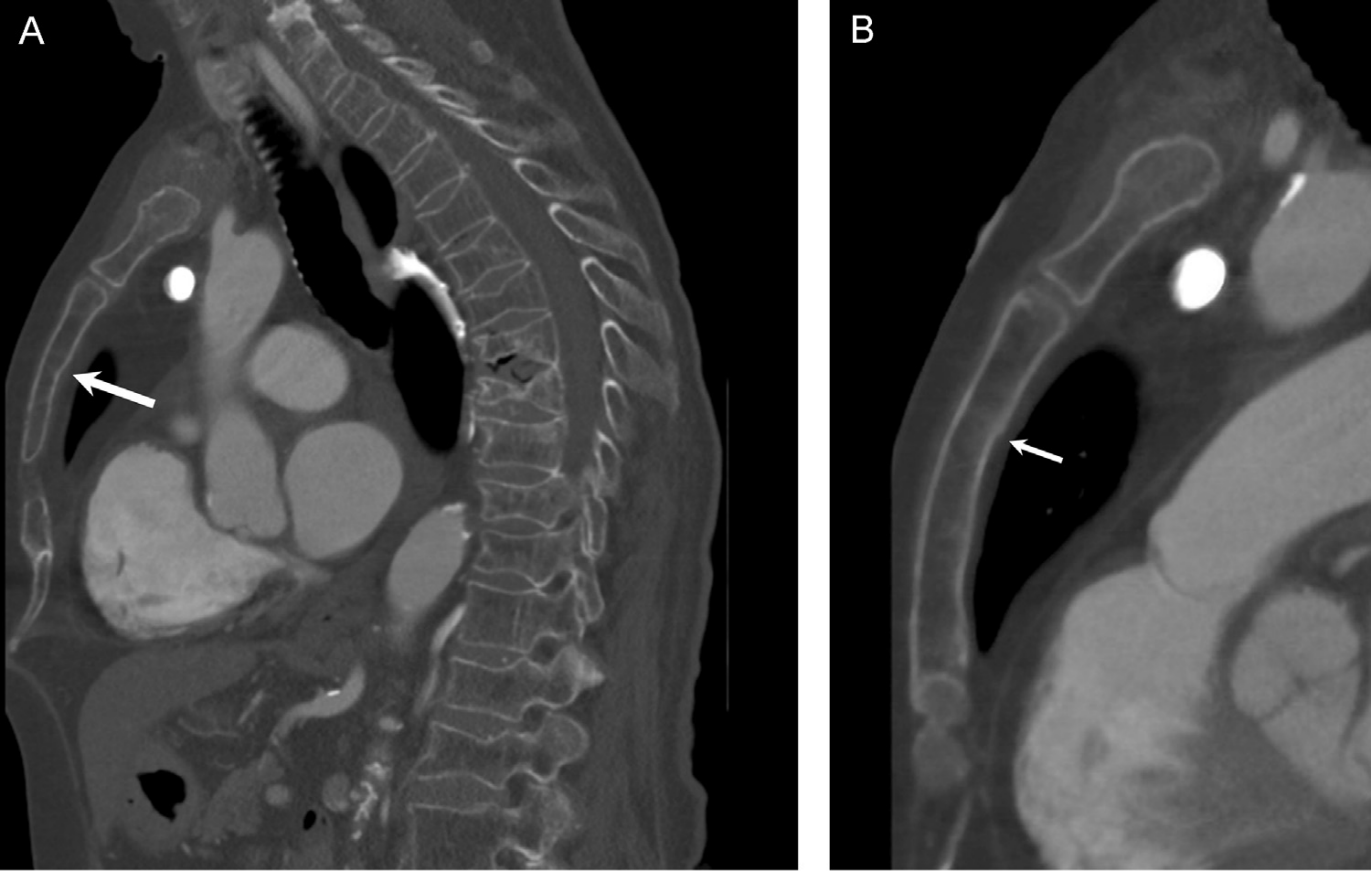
(A) Sagittal CT image of the chest with bone windows shows multiple thoracic compression fractures. These were chronic when compared to prior imaging. There is irregularity of the posterior margin of the sternum. (B) Sagittal CT image of the chest with attention to the sternum shows subtle cortical step-off at the posterior margin of the sternum, consistent with a nondisplaced fracture (arrow).
